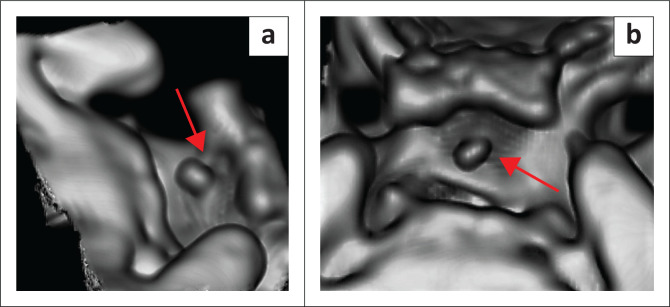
Volume rendered CT of the sella turcica, (a) oblique view and (b) superior view, demonstrating the sellar spine (arrow) protruding into the pituitary fossa.
